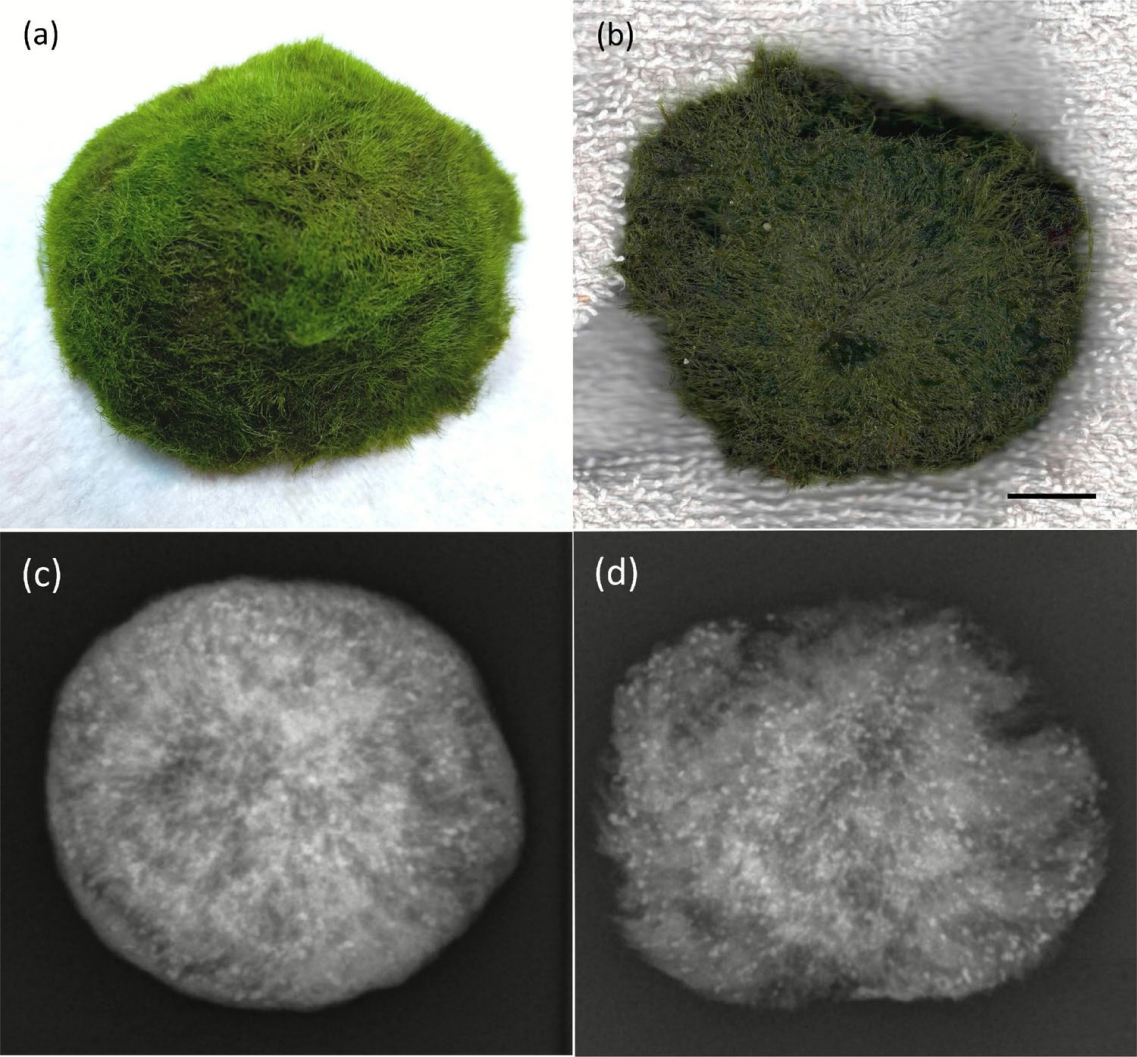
Marimo balls of ~ 50 mm diameter. (a) Photograph of plan view; (b) flat-bed scan of cross-sectioned body; (c) X-ray of whole body; (d) X-ray of cross-sectioned body (DR 600, Agfa HealthCare Ltd, BE). Scale bar: 10 mm
